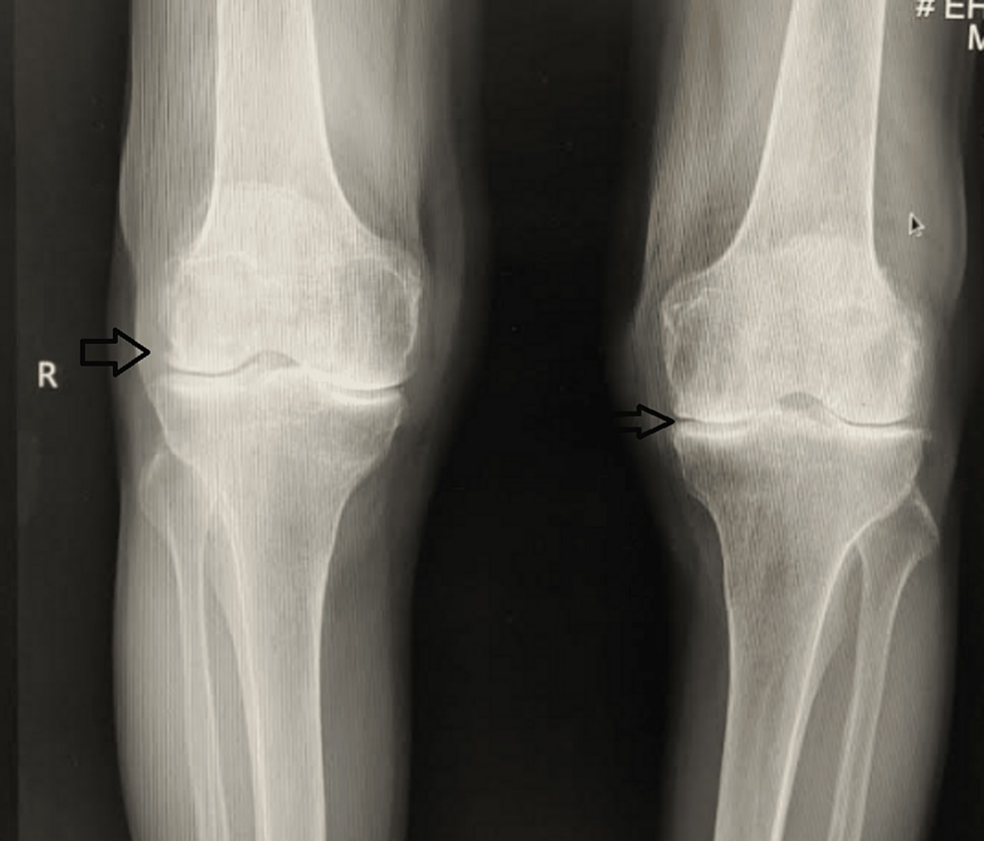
Anteroposterior view plain radiography of both knee joints suggesting features of arthritis (joint space narrowing and osteophytes).
